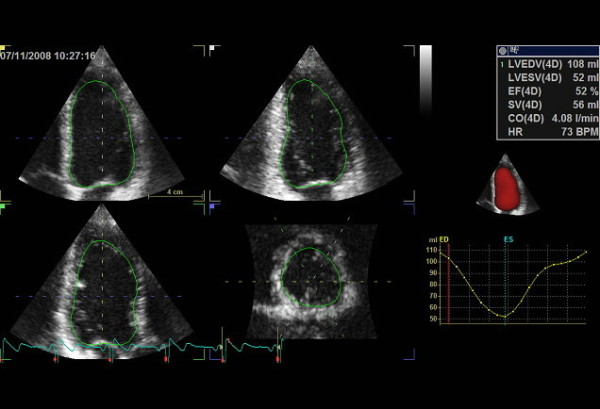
Quad view presentation of left ventricular using 4D auto LVQ software for measurement of left ventricular volumes and ejection fraction with three-dimensional echocardiography. Volume time-plot and quantitative analysis and three-dimensional model are presented in the right panel.
